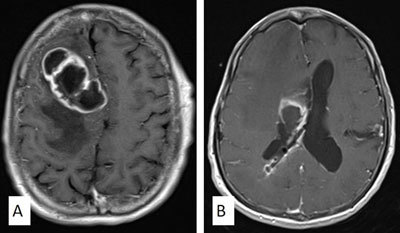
Axial post-contrast T1-weighted MRI of the brain reveals rim-enhancing collections in the right frontal lobe (A) and the right lateral ventricle (B). In addition, there is linear enhancement along the right parietal approached ventricular catheter. These findings are compatible with shunt-related ventriculitis and cerebral abscess.
